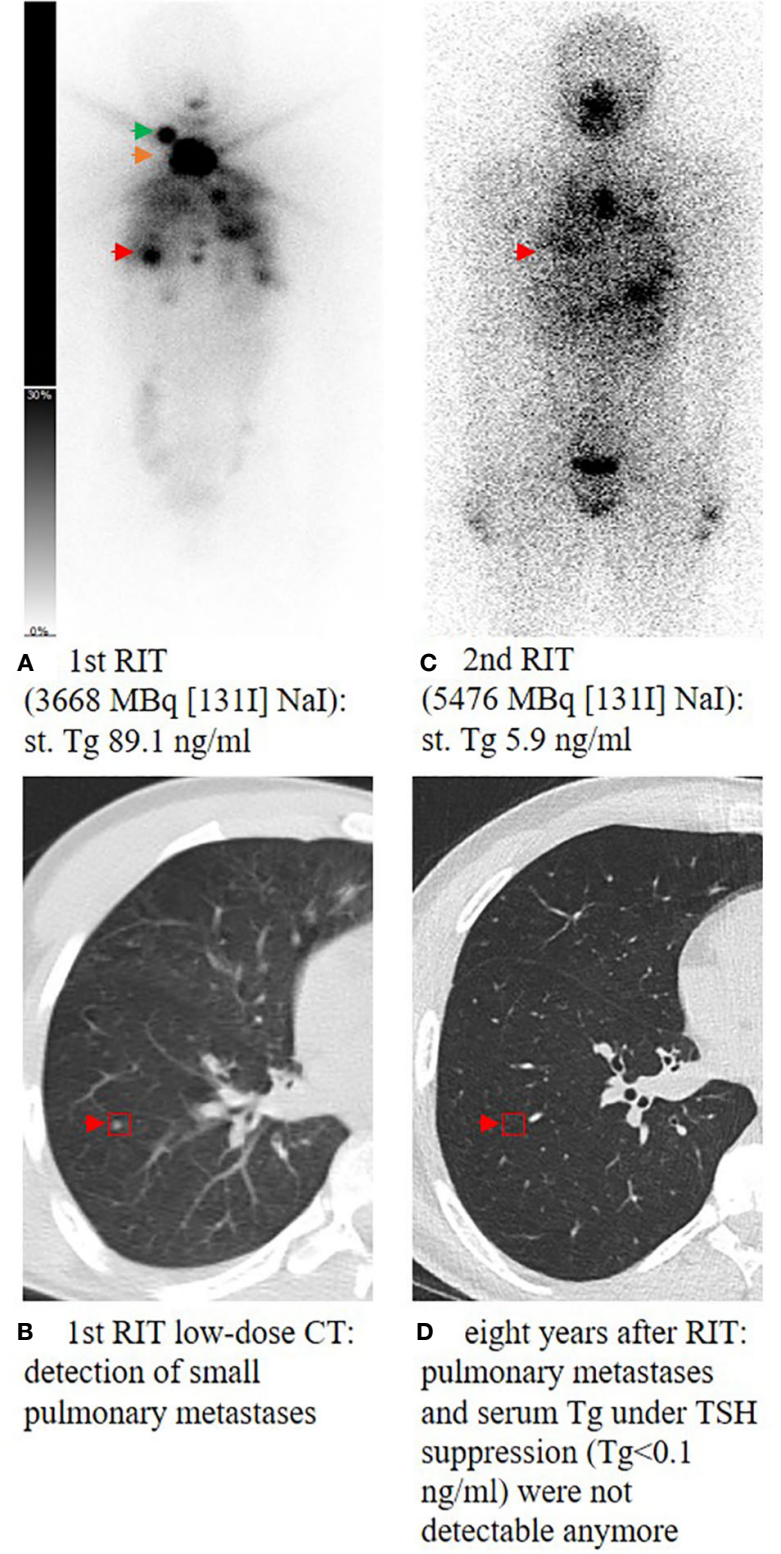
Planar whole-body scintigraphy and CT scan after the first radioiodine therapy (RIT) and during follow-up. A 14-year-old male patient (A) presenting with high residual thyroid bed uptake (orange arrow), an iodine-positive cervical lymph node metastasis in level II on the right side (green arrow), and multiple iodine-positive pulmonary metastases (red arrow) (B). Post-therapeutic scan of the second RIT showed ablation of the remnant thyroid tissue and significant uptake of pulmonary metastases (C). During follow-up, TSH was suppressed consequently; 1.6 years after the last RIT, Tg decreased to <0.1 ng/mL and pulmonary metastases could not be detected anymore in the subsequent CT scans (D). At the last visit, 8 years after the first RIT, the patient still presented with excellent response with Tg <0.1 ng/mL and no remarkable findings in computer tomography of the lung. st., stimulated; Tg, thyroglobulin; RIT, radioiodine therapy; TSH, thyroid-stimulating hormone.
